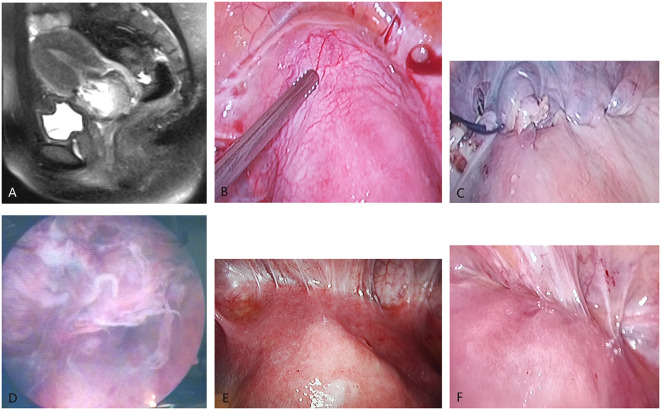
Imaging manifestation of cesarean scar pregnancy (CSP). (A) The sagittal MRI shows that the gestational sac attached to the scar of the lower uterine segment. (B) The laparoscopy directly shows the CSP lesions (there were abundant blood vessels around uterine scar and hemoperitoneum). (C) The appearance of CSP lesions after removal by laparoscopic surgery. (D) The appearance of CSP lesions after removal by hysteroscopy. (E) The appearance of the uterus in the abdominal cavity under direct vision of laparoscopy in hysteroscopy–laparoscopic surgery (↑ indicates the protuberant CSP lesions). (F) The appearance of the uterus after the lesions were removed by hysteroscopy under the direct vision of laparoscopy during the hysteroscopy–laparoscopic surgery (the protuberant CSP lesions disappeared).
